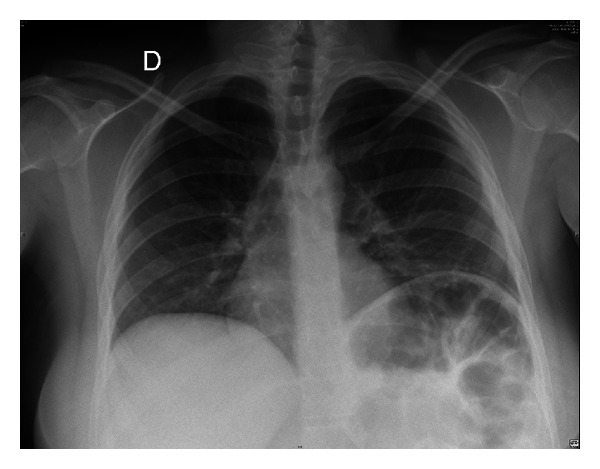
Chest X-ray showing left hemidiaphragmatic elevation due to the accumulation of gas in the splenic angle of the colon.
